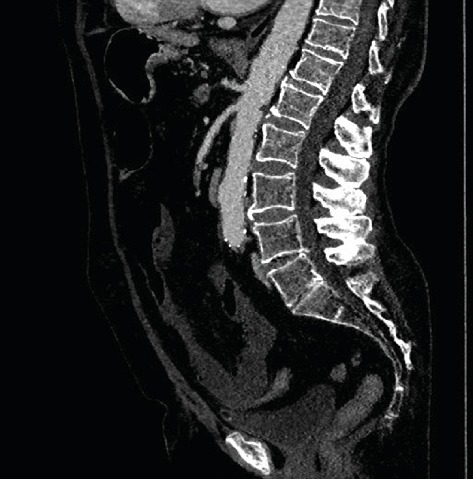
Computed tomographic angiography (angio-CT) of abdominal blood vessels in a patient with acute mesenteric ischemia of embolic cause. A clot obstructing the vessel lumen is visible.
